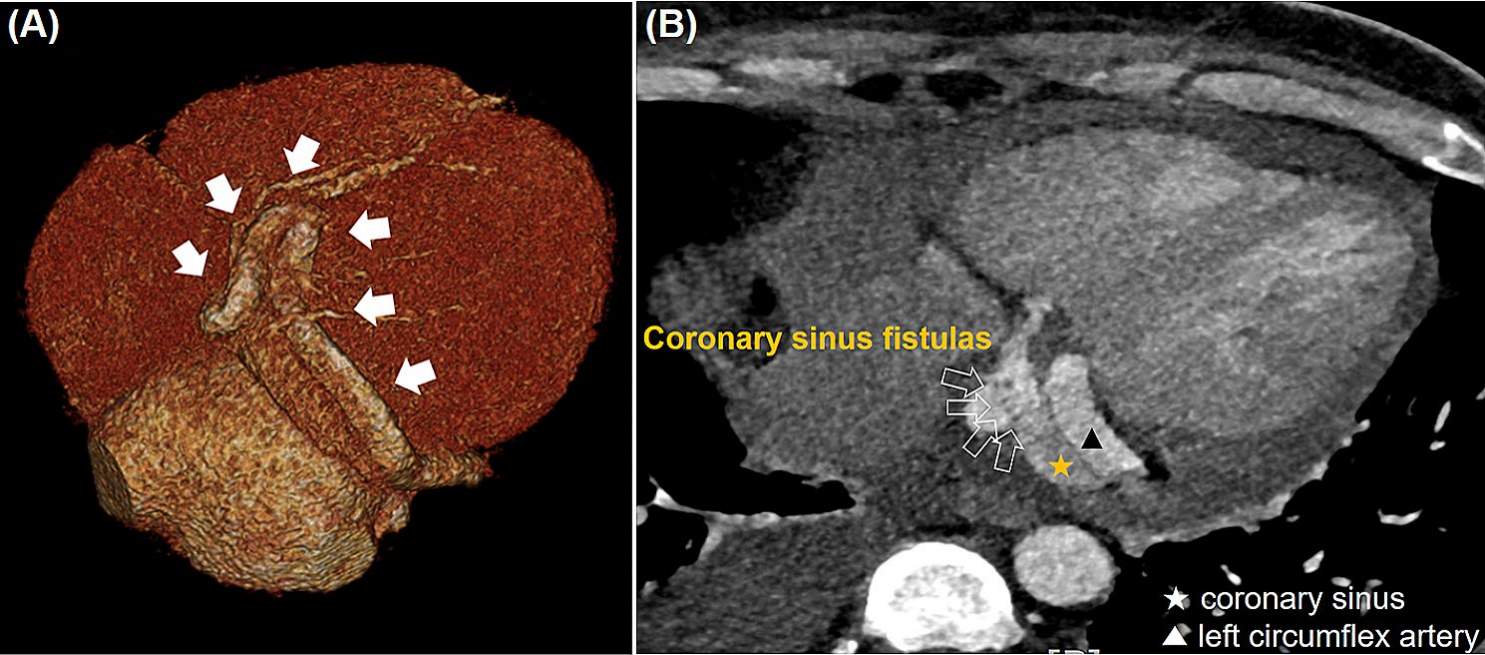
Cardiac computed tomography indicated that (A) LCx (white solid arrow) was enlarged to an overall diameter of approximately 1.2 cm while (B) multiple left circumflex CAFs (white blank arrow) draining into CS were identified
